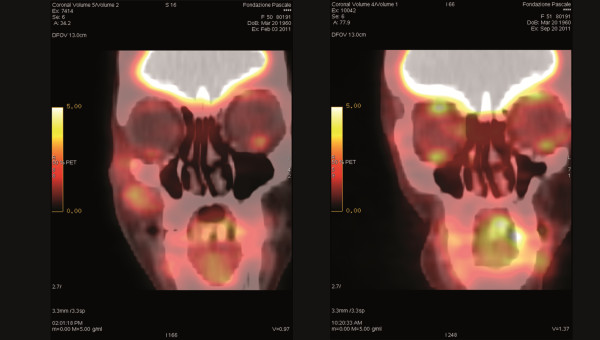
(Left) Post electrochemotherapy and (right) post surgical treatment FDG-PET scan with SUVmax respectively decreased to 5 and to 1,3.
